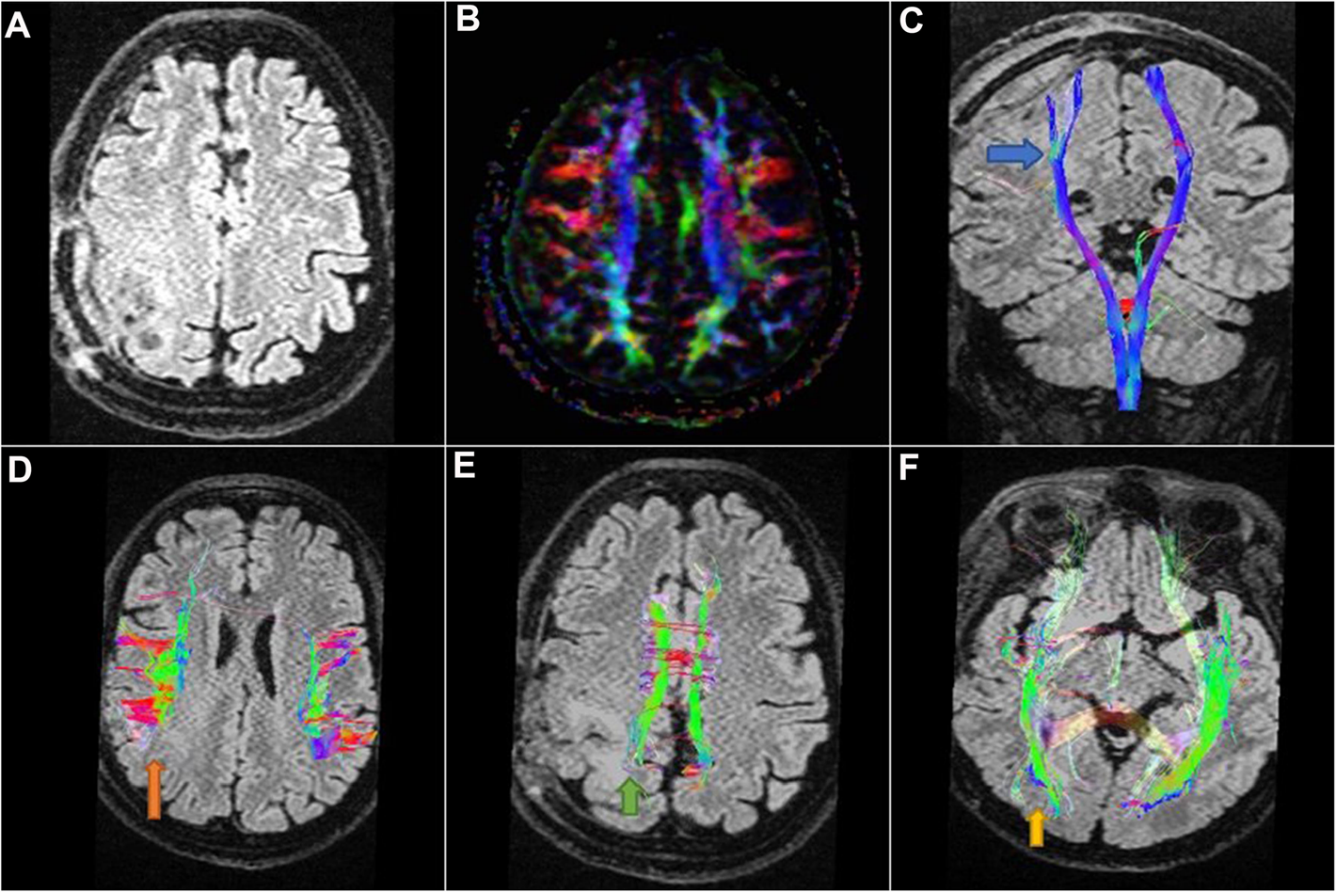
Case number 1. Postoperative radiological examination of Fig. 1 patient. a, b Axial FLAIR WI and DTI color-coded map revealing right parieto-occipital craniotomy flap with right parietal postoperative changes, no residual masses. c Sagittal FLAIR WI with 3D reconstruction of the corticospinal tracts revealing normalization of position and anisotropy of the splayed tracts (blue arrow). d Axial FLAIR WI with 3D reconstruction of superior longitudinal fasciculus (orange arrow). e Axial FLAIR WI with 3D reconstruction of the cingulum (green arrow) revealing normalization of anisotropy. f Axial FLAIR WI of inferior longitudinal fasciculus (yellow arrow) revealing normalization of position and anisotropy of their tracts
